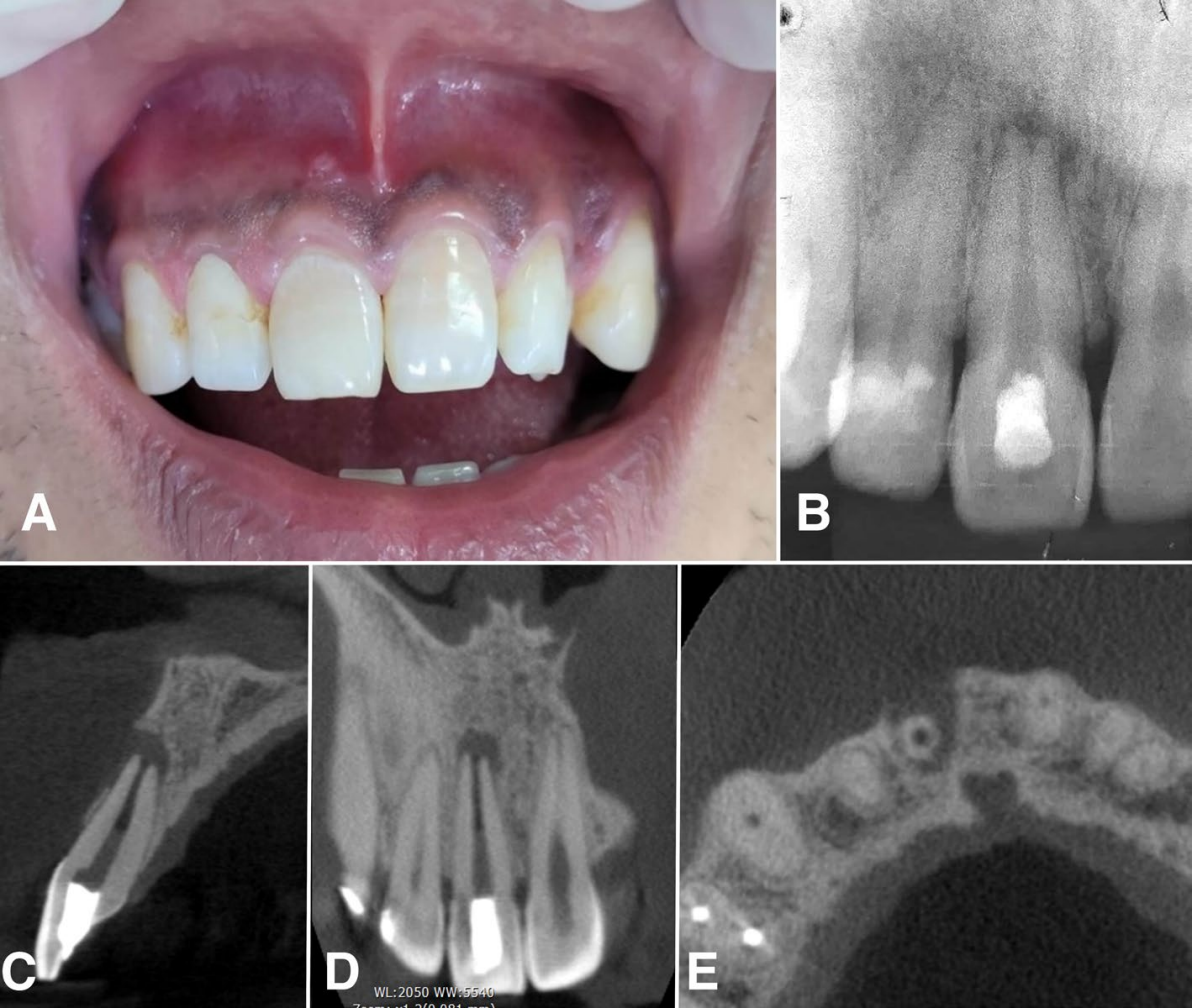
(A) Clinical aspect of maxillary right central incisor (tooth #11, FDI notation). A sinus tract is also visible a sinus tract on the apical gingiva. (B) Periapical radiograph of tooth #11 showing temporary restoration, apical lesion, and open apex. Preoperative CBCT sagittal (C), coronal (D), and axial views (E), showing loss of the buccal cortical plate (C and D), incomplete root formation, and apical lesion (C, D, and E).
